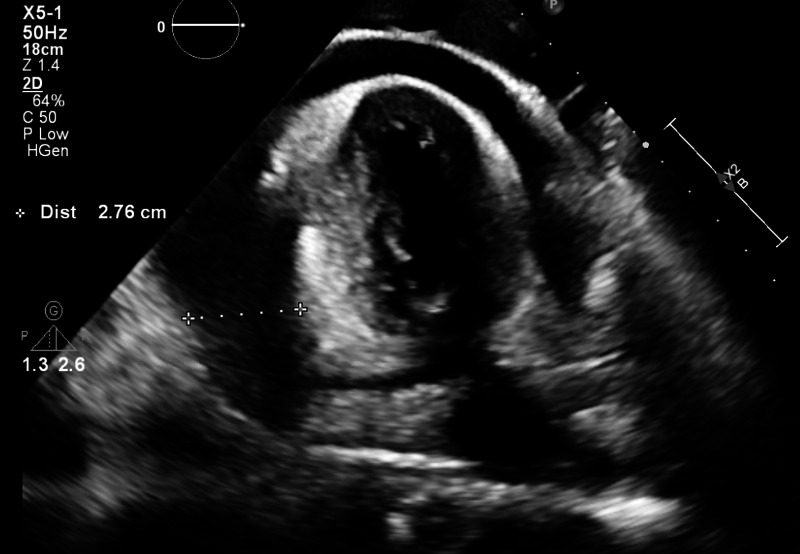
Transthoracic echocardiogram showed normal left ventricular function and large circumferential pericardial effusion.
